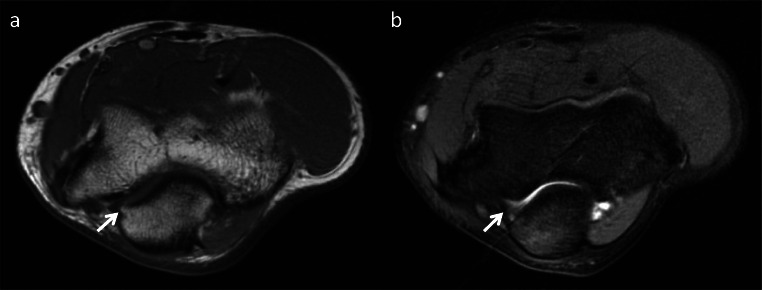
a Axial T1 MR image of the elbow demonstrates a small osteophyte along the posteromedial olecranon (arrow). b Axial T2 MR image demonstrates posteromedial ulnohumeral synovitis (arrow), mild pericapsular edema, and bone marrow edema-like signal in the olecranon
